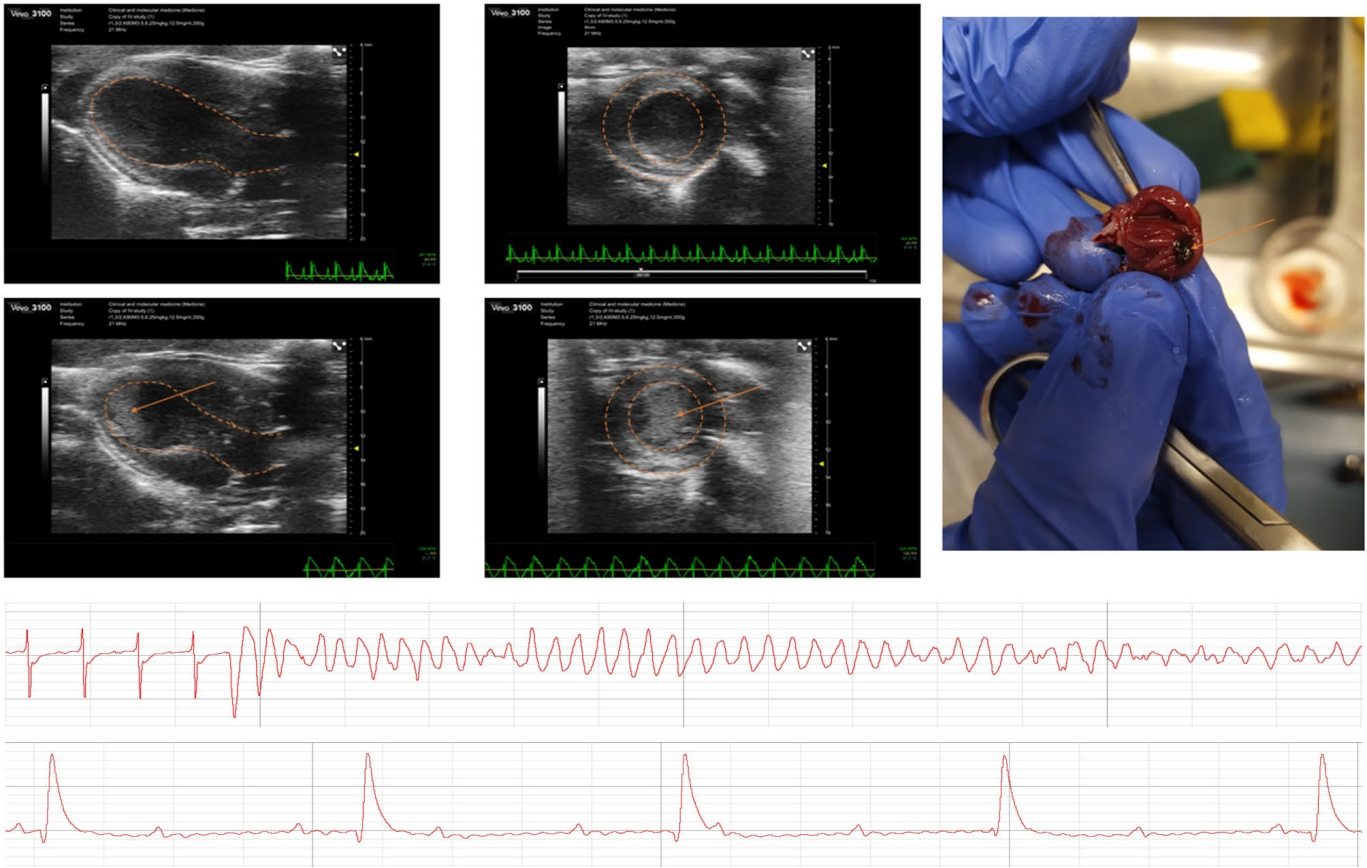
Complications Reproduced in TS Model. (A) Thrombus formation is shown in echocardiographic images taken 8 h post-induction (bottom) in the LAX view, showing visible apical ballooning (bottom left). This was confirmed in the SAX view, 9 mm below the mitral valve (bottom right), and via post-mortem analysis (right). Echocardiographic images from 3 h post-induction (top) show extensive apical ballooning without thrombus. The endo-/epicardium (dashed line) and thrombus (arrow) are highlighted for clarity. (B) Ventricular arrhythmia occurred early in the induction phase, and was the second most frequent cause of death after acute heart failure. (C) Atrioventricular blockage of varying degrees was observed. Here, a third-degree blockage is shown, marked by the total disconnection between atria and ventricles.
